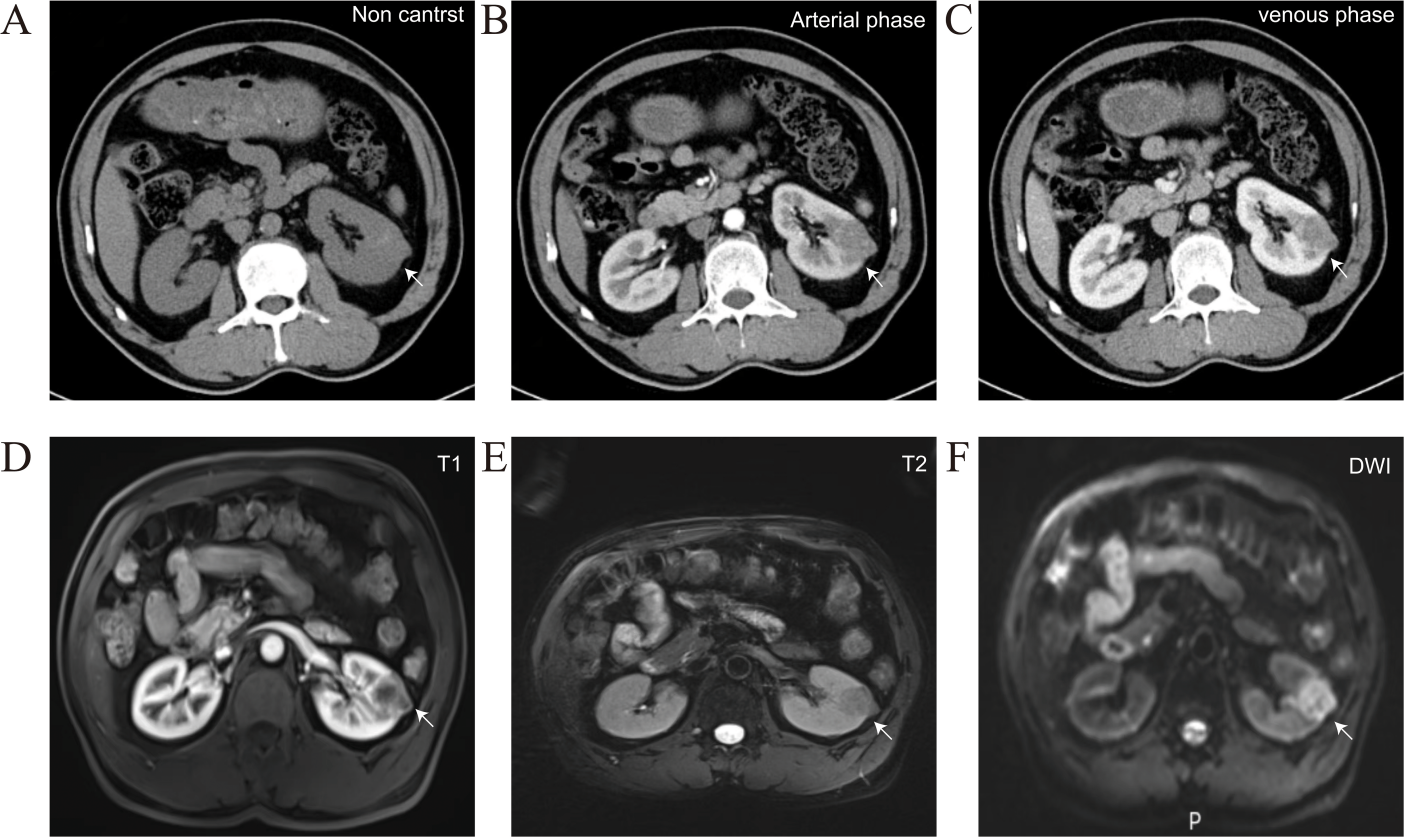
Representative CTU and MRI images of the patients, arrows indicate the location of the tumor. (A) Non contrast CTU scan showed irregular low-density shadow with punctate high-density foci, and its boundary was unclear. (B, C) Arterial phase and venous phase CTU scan showed irregular and inhomogeneous enhancement. (D-F) MRI scan confirmed a hypovascular tumor in the left kidney with heterogeneous delayed-phase enhancement.
